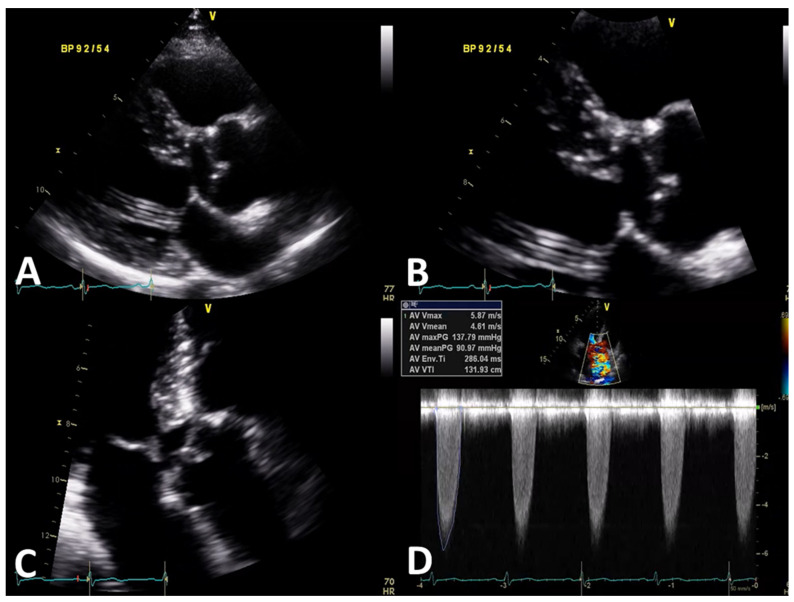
Transthoracic echocardiographic image showing a parasternal long-axis view (A,B), apical four-chamber view of the aortic valve (C), and continuous-wave Doppler across the aortic valve (D).
